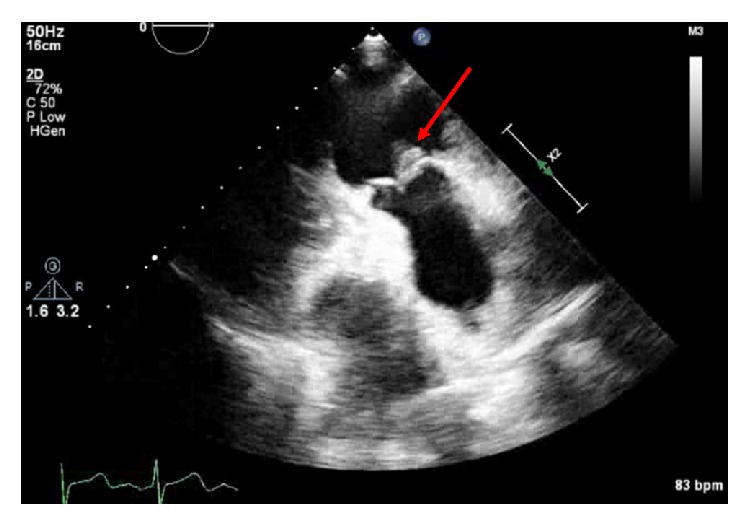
Transthoracic echocardiogram showing a 10 mm × 11 mm vegetation (arrow) on the ventricular aspect of the pulmonic valve.
